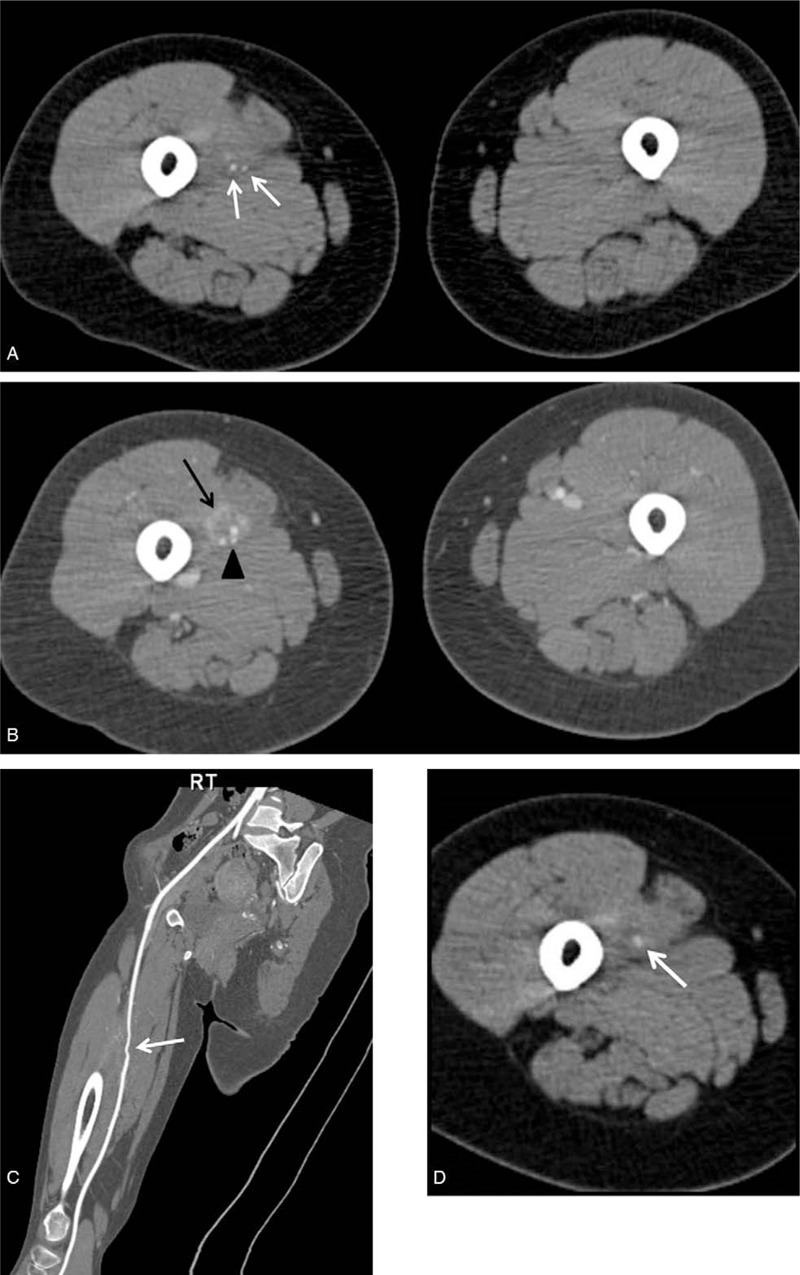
Computed tomography (CT) images obtained at the general surgery clinic 1 year before referral and at 12-month follow up. (A) Before contrast enhancement, focal calcification (white arrows) was observed in the medial aspect of the right thigh. (B) Following contrast enhancement, obliteration of the vein was observed with replacement by the mass, which showed peripheral enhancement (black arrow). Calcification was located in the center of the lesion (black arrow head). The diameter of the artery was reduced compared to that of the left side. (C) Focal narrowing of the artery was verified in vessel reconstruction images. (D) CT imaging at 12 months follow-up showing absence of calcification that was observed previously while new focal calcification could be seen.
